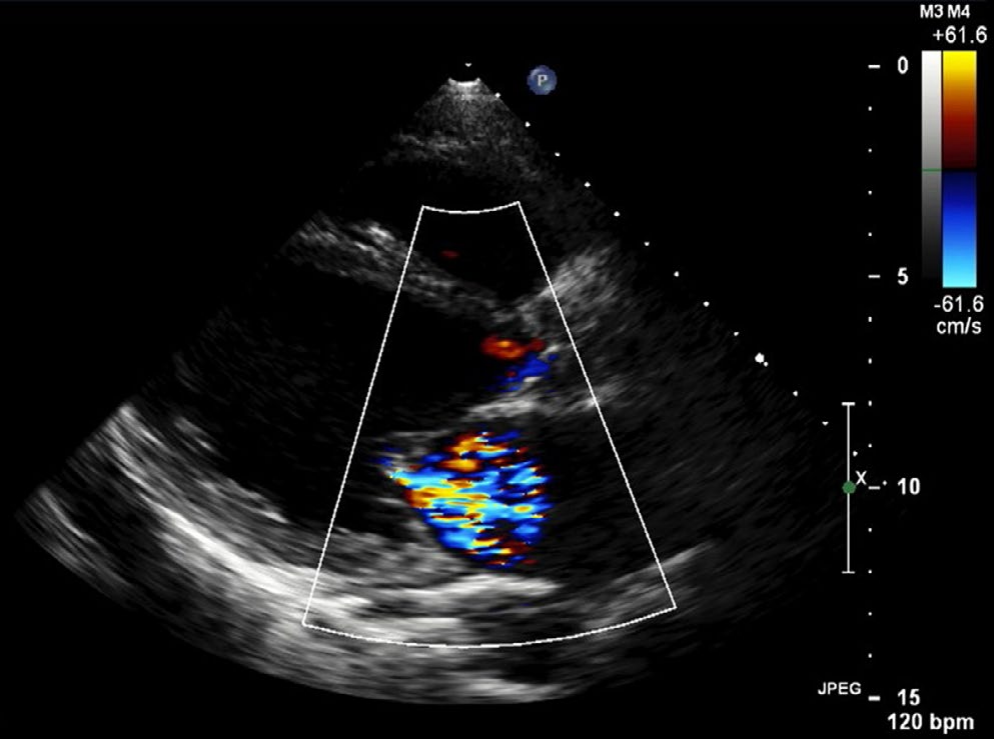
Long‐axis view on echocardiography on admission. Echocardiography showed diffuse severe hypokinesis, increased left ventricular end‐diastolic diameter, and moderate mitral regurgitation
